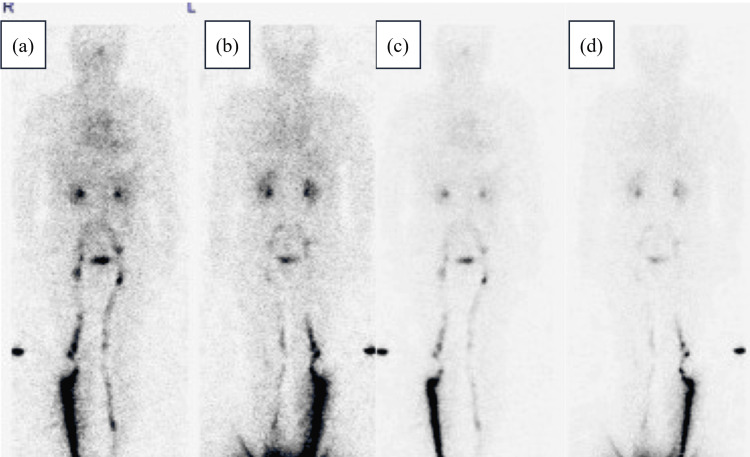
Lymphoscintigraphy on the 10th hospital day Lymphoscintigraphy performed immediately (a) anterior view, (b) posterior view, and 30 minutes later (c) anterior view, (d) posterior view. The imaging shows no obvious lymphatic leaks.
